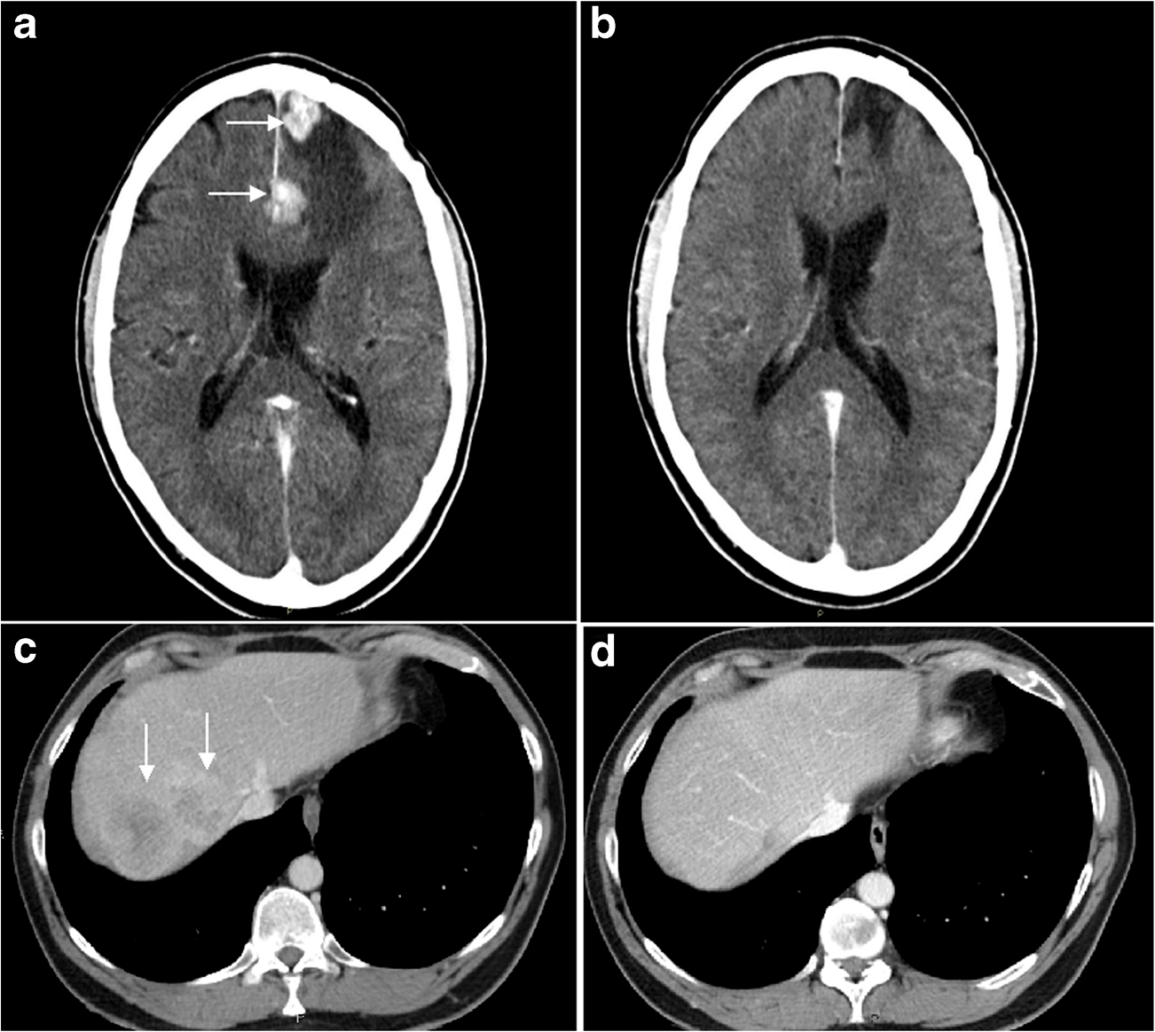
CT-scans from a patient with CNS and liver metastases 4 months after start of ilixadencel treatment but before additional treatment with sunitinib (A and C, respectively) and 6 months (B) or 12 months (D) after start of sunitinib treatment. The data in figure originates from Laurell *et al*. [46].
